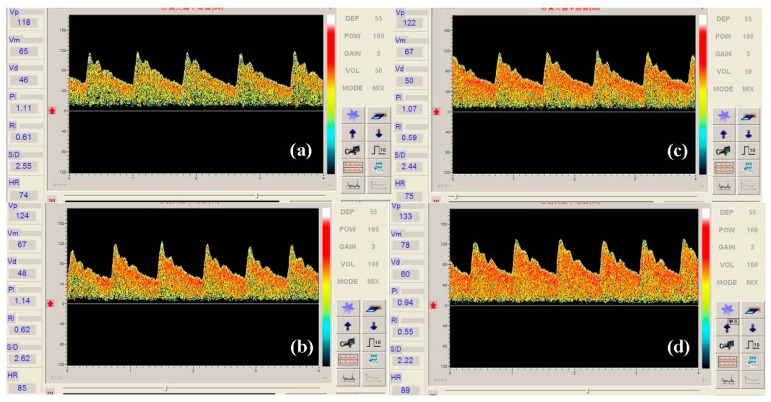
Doppler sonographic measurement of MCA at the transmission powers of (a) 2.6 J; (b) 11.12 J; (c) 15.5 J; and (d) 19.8 J.
